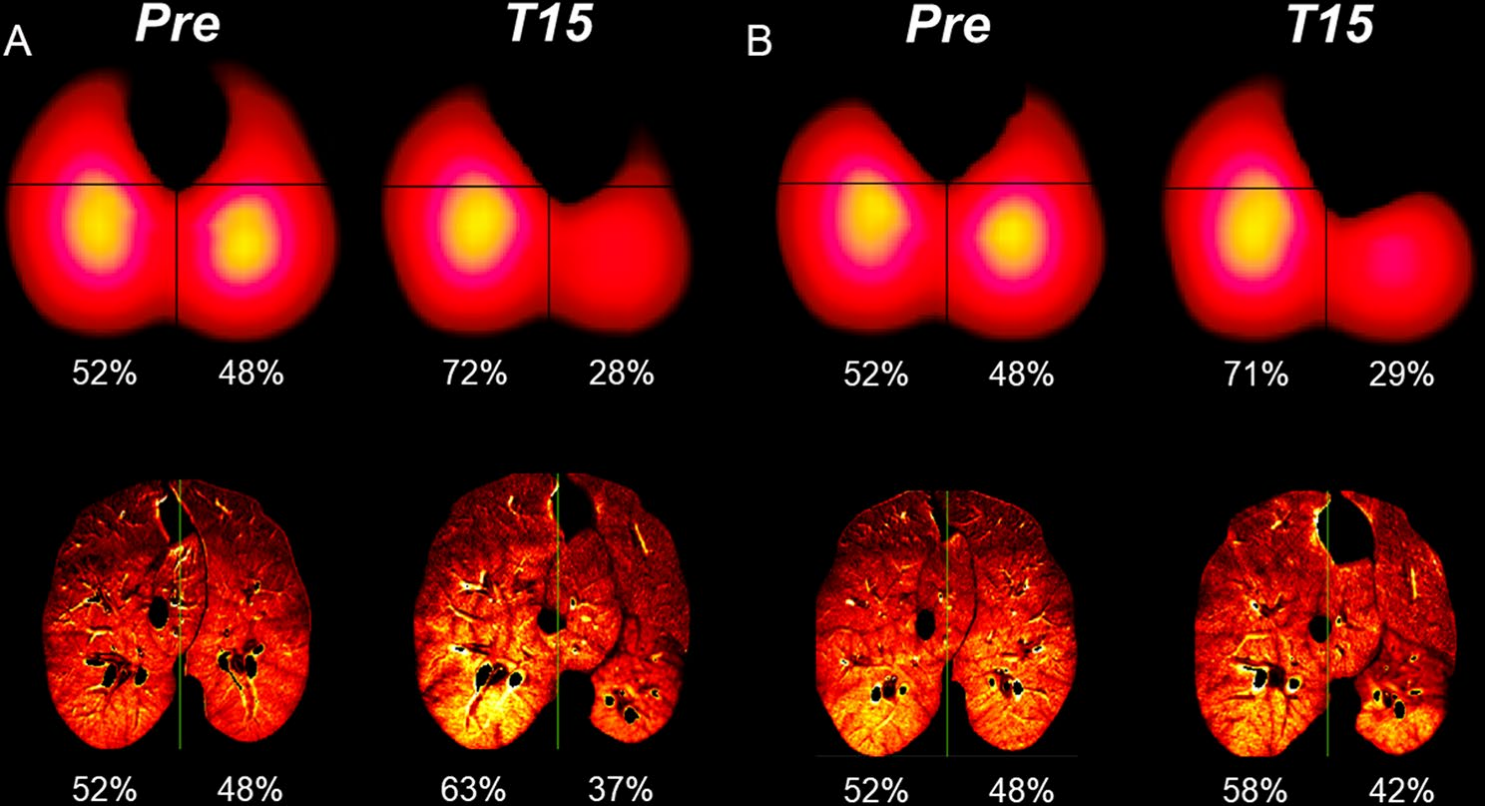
Perfusion distributions. Electrical impedance tomography (EIT) and dynamic contrast-enhanced computed tomography (CT) perfusion distributions with *Balloon* (A) and *Valves* (B) under fraction of inspired oxygen 1.0. *Pre* (under the *standard mechanical ventilation settings*) and *T15* (15-minute occlusion). The EIT and CT images were sub-segmented into two regions-of-interest (ROIs): right and left hemithoraces. Both imaging techniques showed a decreased perfusion in the left ROI at *T15*
